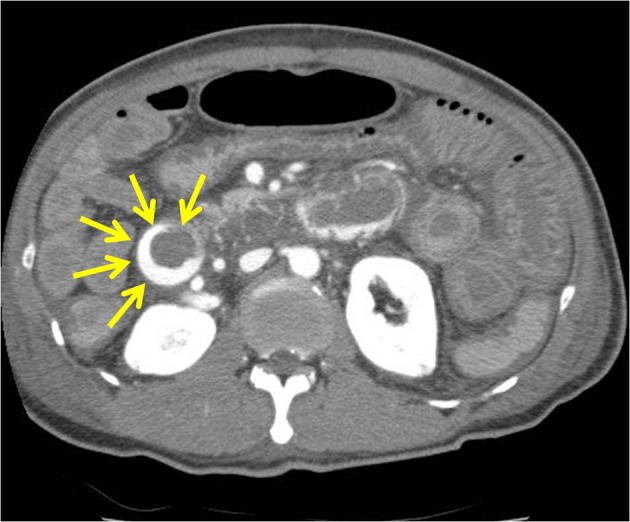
High attenuated lesion is observed at the third part of duodenum in the arterial phase of the abdominopelvic computed tomography scan (arrows).
